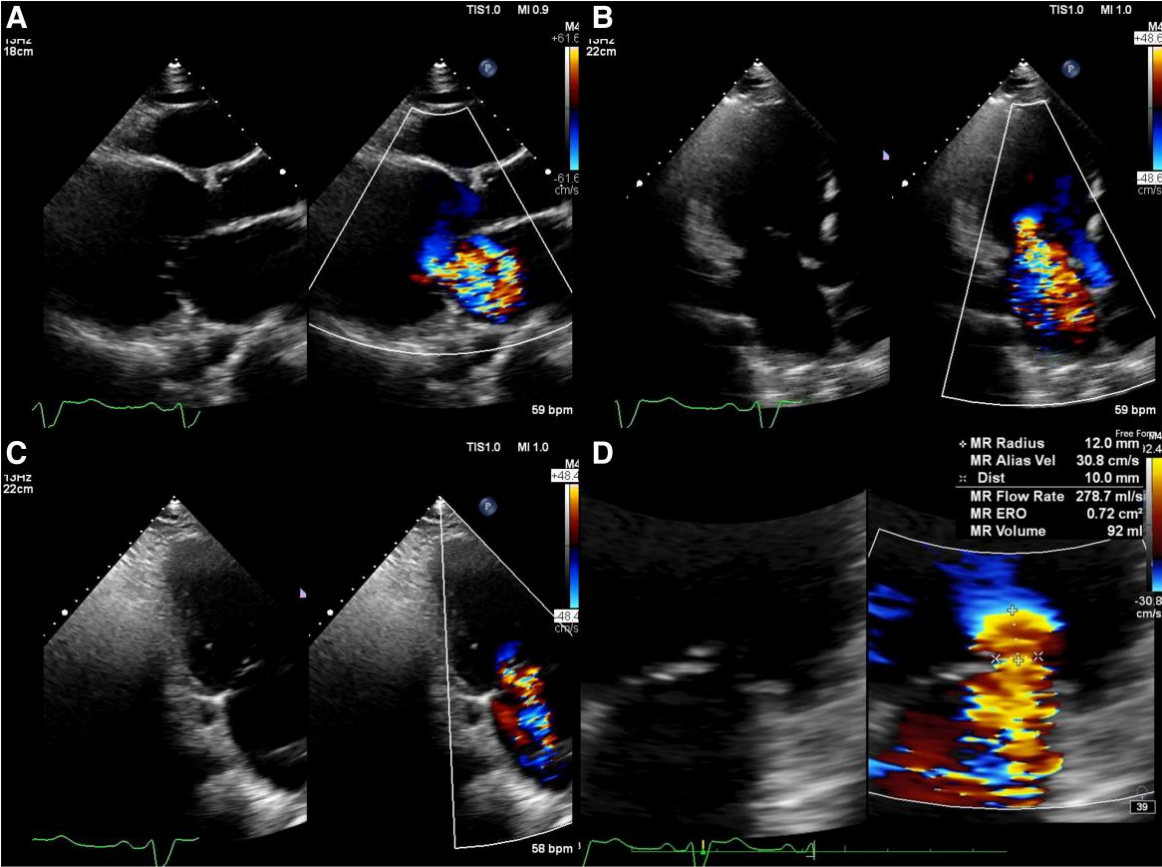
Preprocedural transthoracic echocardiography images show severe mitral regurgitation from A2/P2 (*A–C*). The quantification of mitral regurgitation by the Proximal Isovelocity Surface Area method showed an effective regurgitant orifice area of 72 mm^2^ and a regurgitant volume of 92 mL (*D*).
